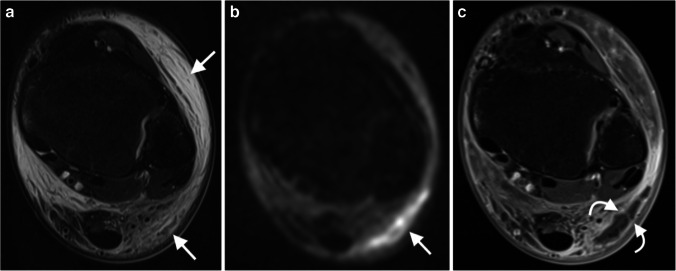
A 12-year-old girl with a soft-tissue abscess associated with arthritis and cellulitis of the ankle. a Axial STIR shows ill-defined and circumferential oedema of the soft tissues around the ankle (*arrows*). b Axial DWI (b value of 800 s/mm^2^) allows delineation of a circumscribed subcutaneous collection (*arrow*). c An axial T1-weighted fat-suppressed image after contrast injection confirms a subcutaneous abscess (*curved arrows*). DWI diffusion-weighted imaging, STIR short tau inversion recovery
